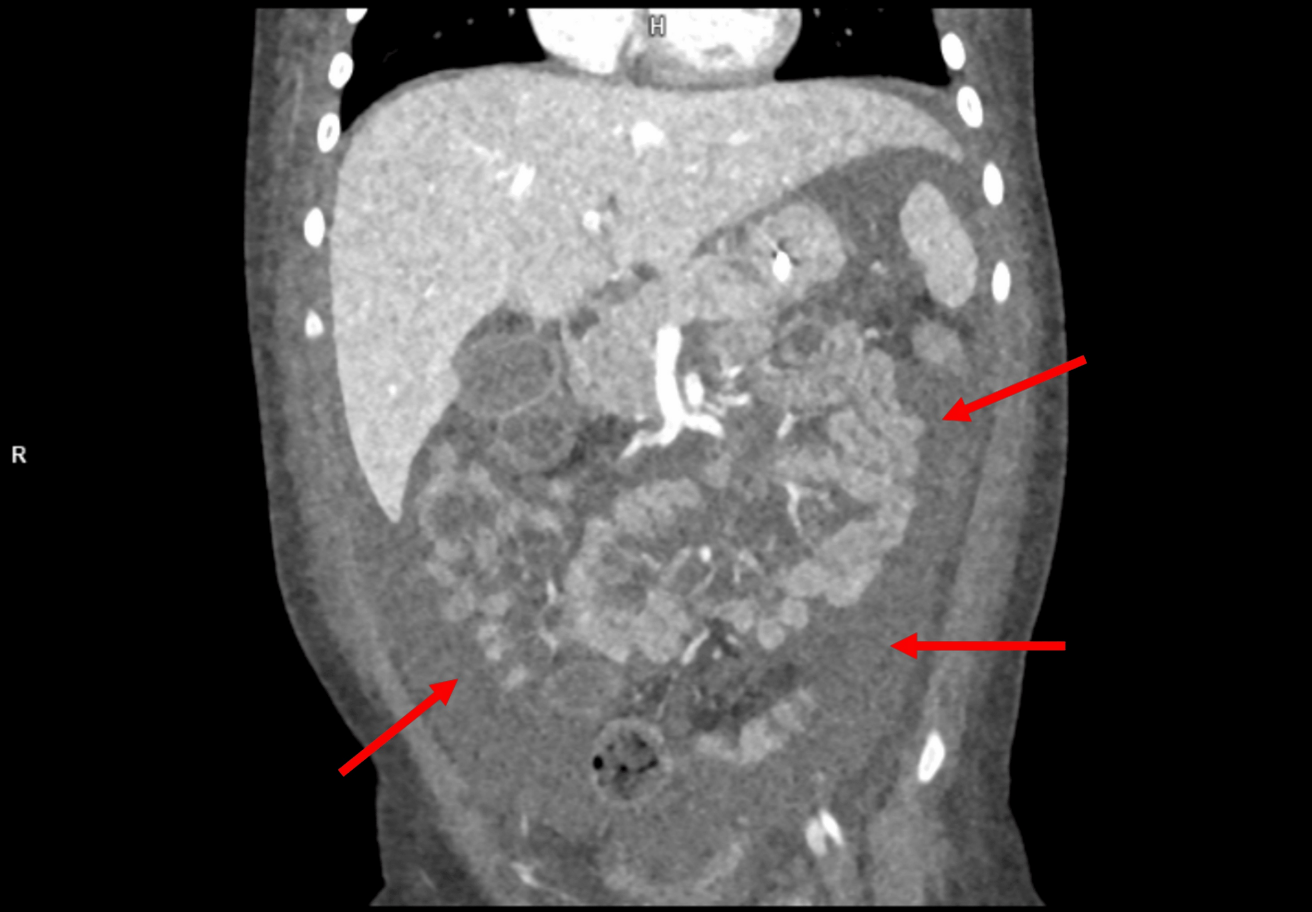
Abdominal CT scan showing diffuse free fluid in the abdominal cavity. The red arrows indicate free abdominal fluid.
